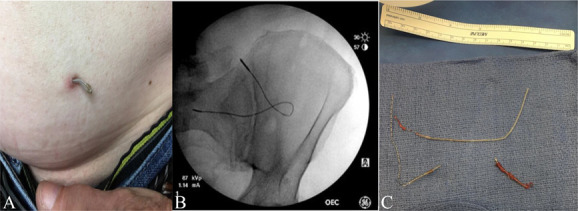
Case 1. (A) Peripheral lead from ilioinguinal nerve stimulator protruding from the skin. (B) X-ray of a peripheral stimulator before removal in OR. (C) Peripheral stimulator lead which fractured during removal.
